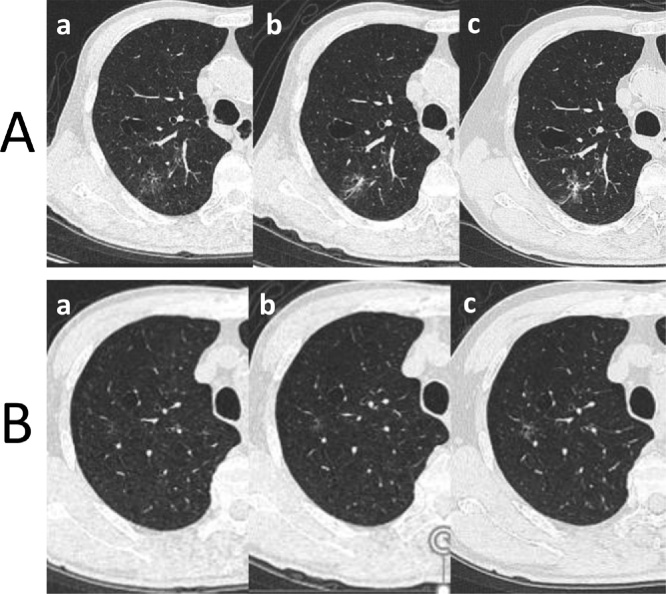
A) The solid component of the GGN in segment 2 had increased in size according to the CT findings. B) Pure GGN in segment 1 adjoining the bulla wall on CT. a. Thirteen months before surgery. b. Nine months before surgery. c. One month before surgery.
